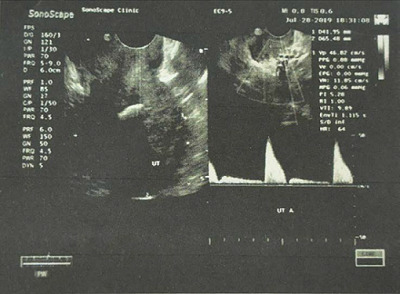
Transvaginal ultrasound showing an intrauterine hyperechogenicity with post-acoustic shadowing suggestive of calcification associated with chronic endometritis.
